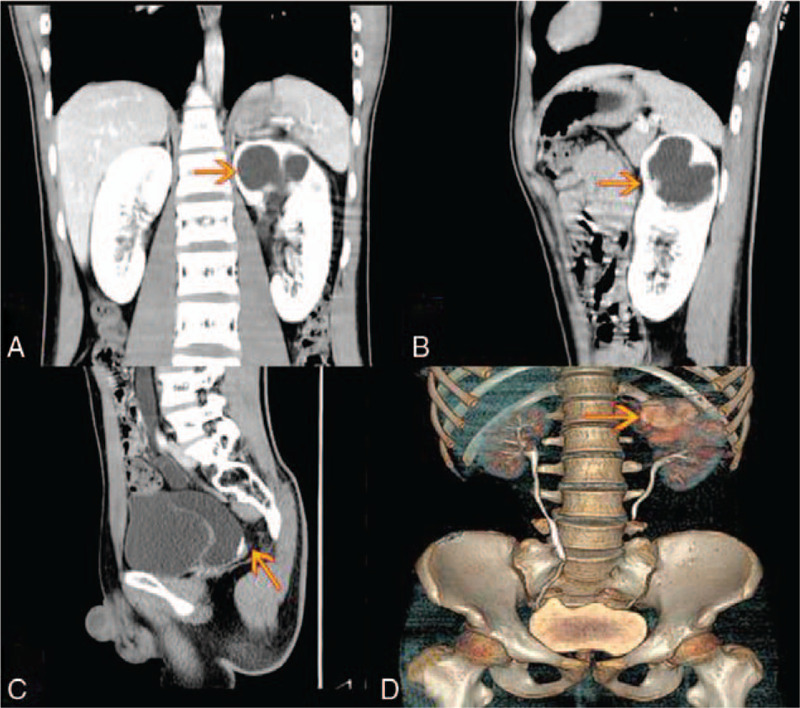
A 16-year-old male teenager with duplicate kidneys. A, Coronal B, Left sagittal contrast-enhanced CT C, Left ureteral lower calculi D, CTU showed the left ureteral duplication malformation (arrow).
